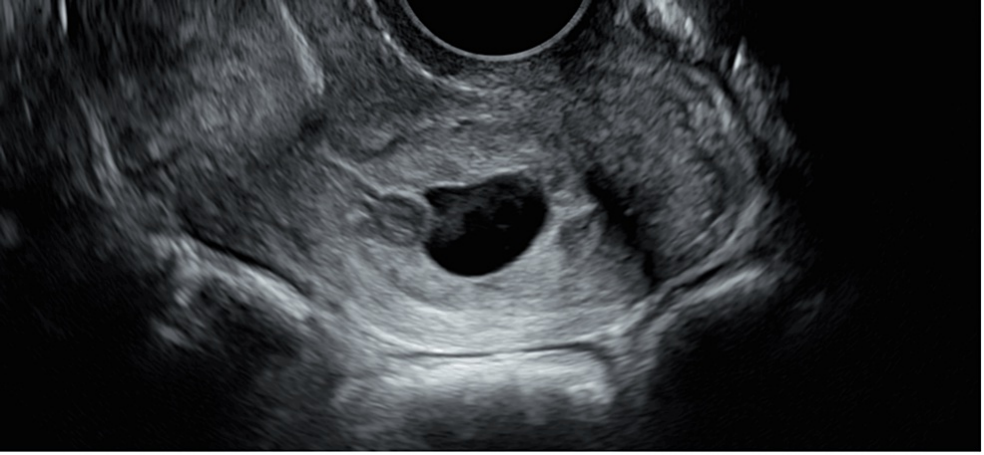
Transvaginal pelvic ultrasound at initial presentation showing a cervical ectopic pregnancy.
